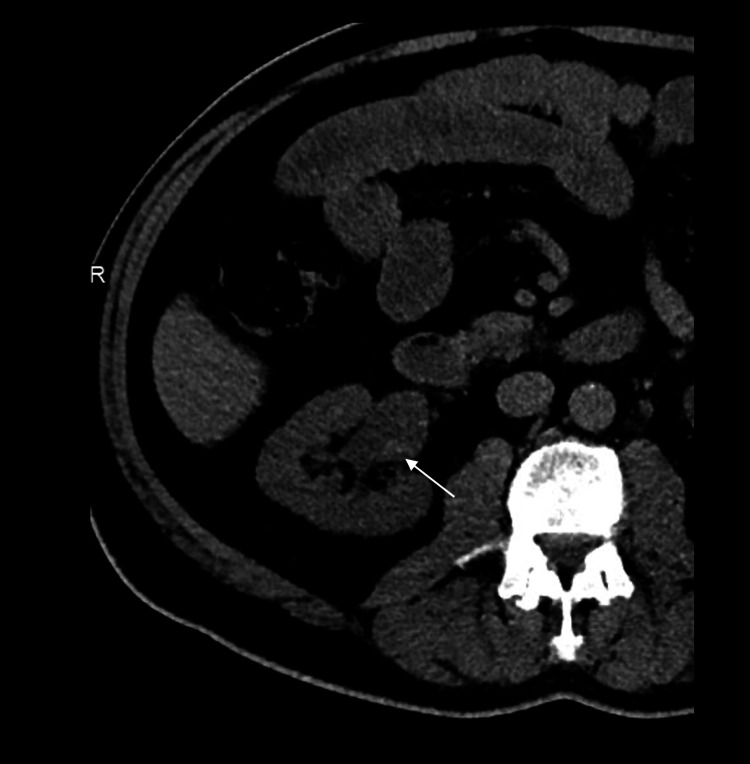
Axial image from the patient’s non-contrast CT revealed interval stent removal and right renal pelvis dilation (white arrow). A subtle area of dependent increased attenuation was noticed retrospectively in the right renal pelvis.
